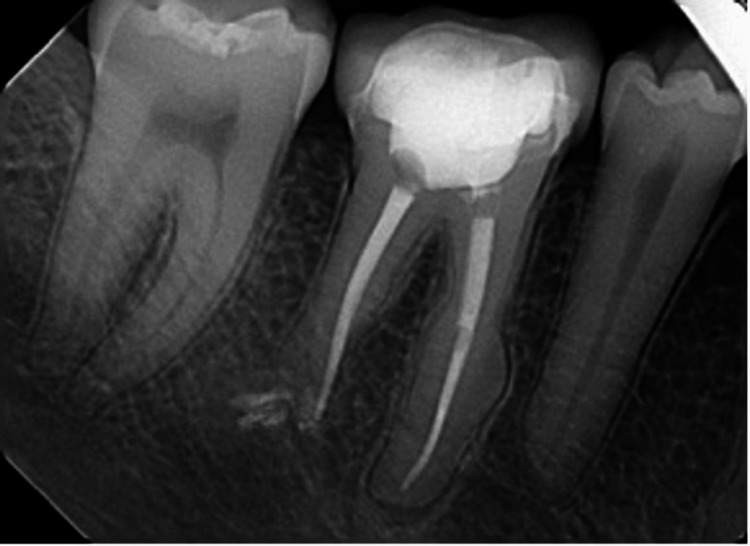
Periapical radiograph after one-year follow-up.
